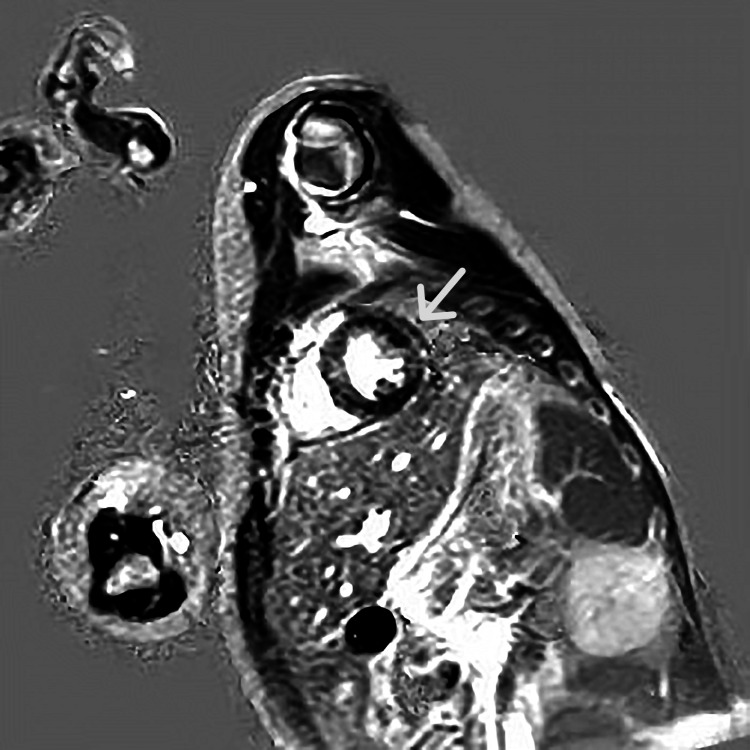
cMRI: Intraventricular septum enhancement. cMRI: Cardiac magnetic resonance imaging
